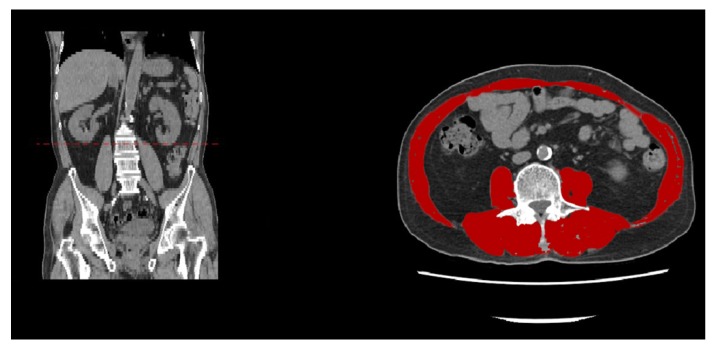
Computed tomography image of the third lumbar vertebral level. The following skeletal muscles are outlined in red: psoas, paraspinal, transverse abdominal, external oblique, internal oblique, and rectus abdominis muscles.
